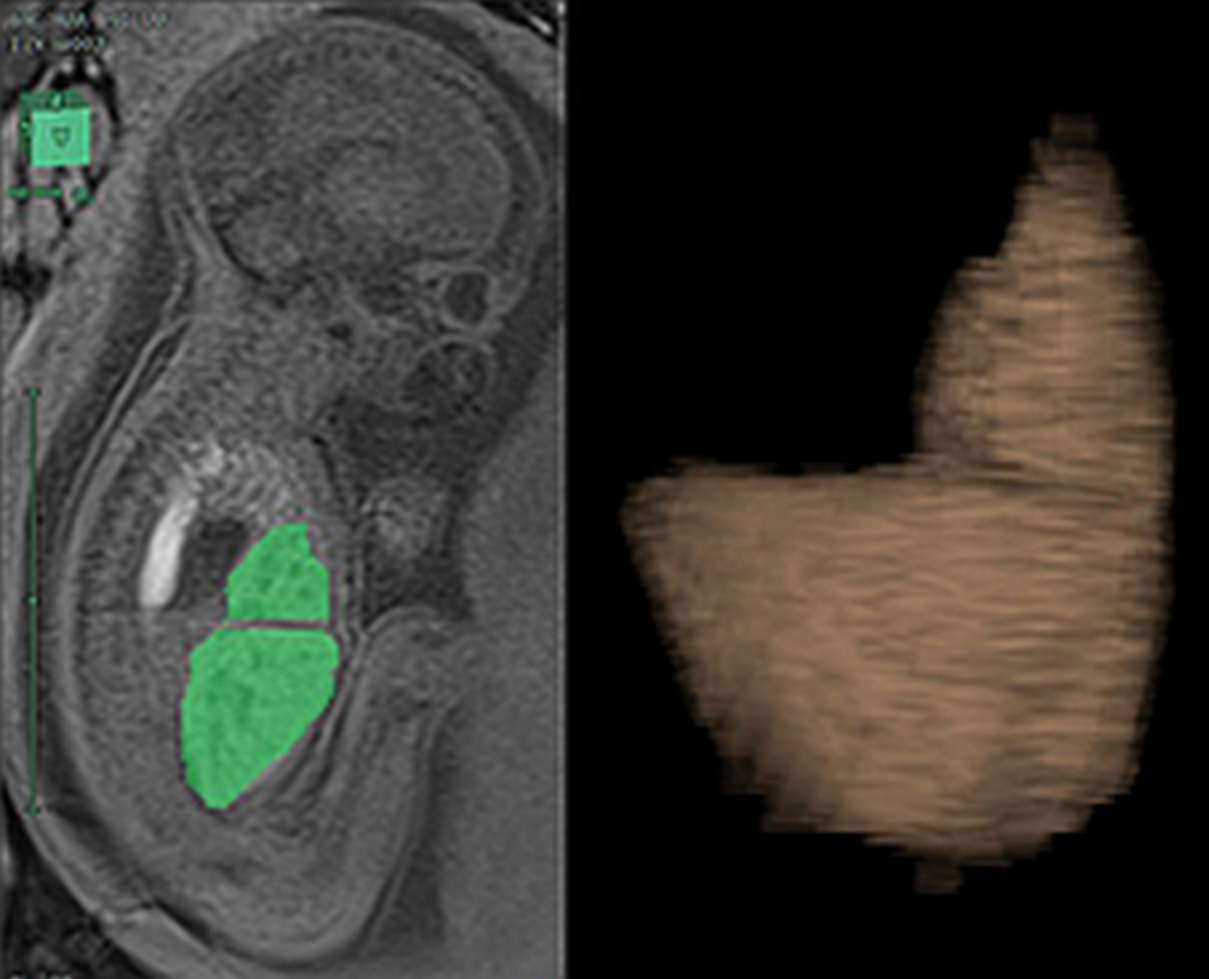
Liver volume assessment. MRI segmentation and 3D reconstruction of the fetal liver with the calculation of the herniated liver on T2-sequences.
